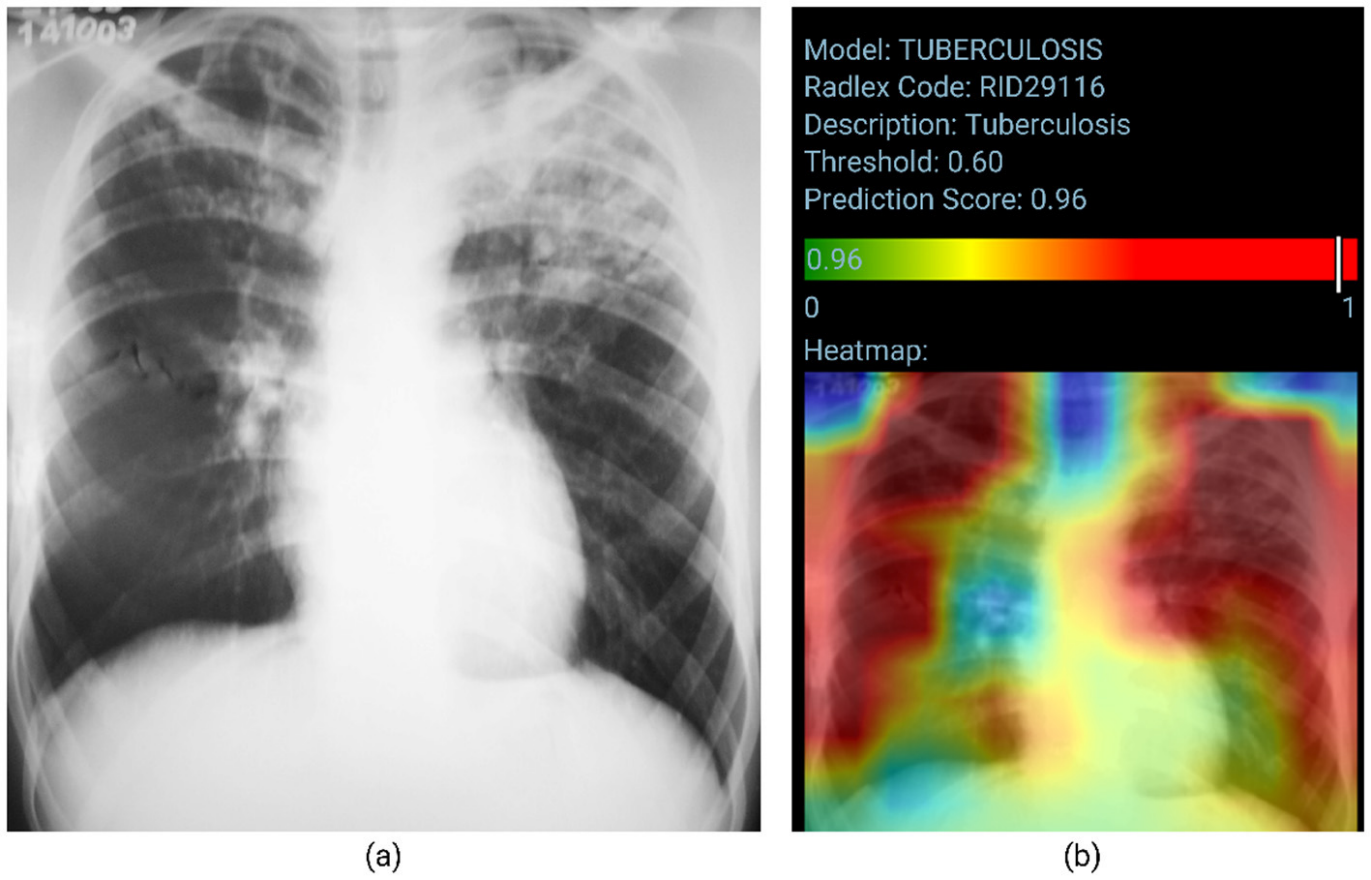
True positive sample for the Tuberculosis Model. (A) Shows the original chest x-ray and (B) shows the TBM result.
